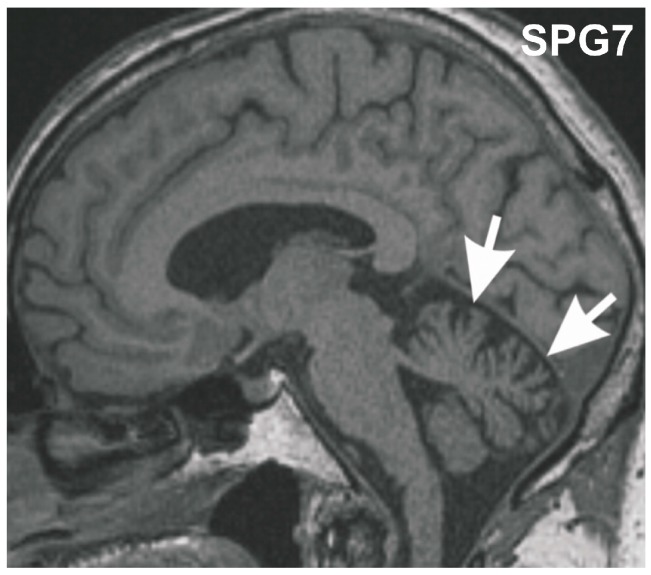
Midsagittal T1 weighted MRI of the SGP7 index patient at age 70 years. The MRI shows mild vermian cerebellar atrophy (arrows).
